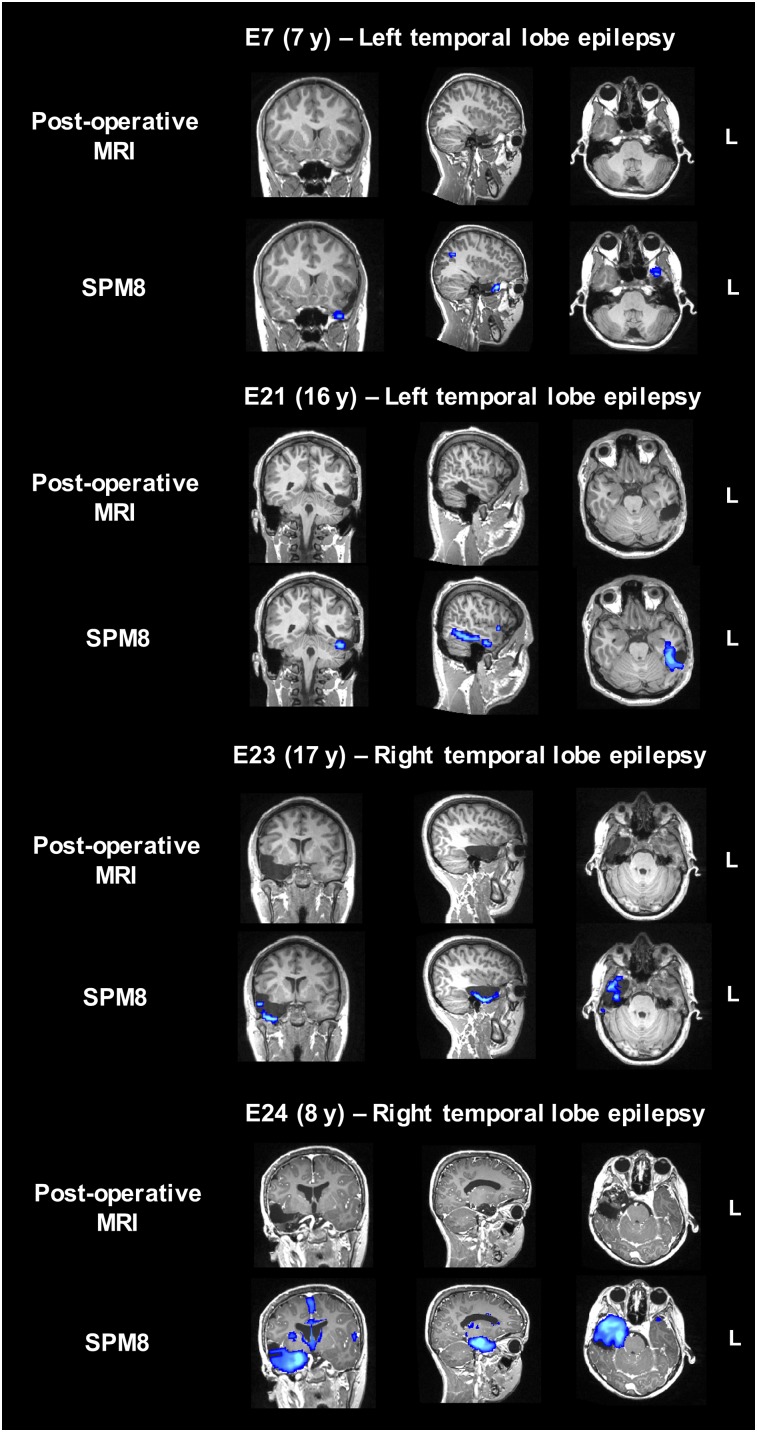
Presurgical SPM8-based statistical maps overlaid on coregistered postsurgical MRI scans for four representative seizure-free patients (patients E7, E21, E23, and E24).
